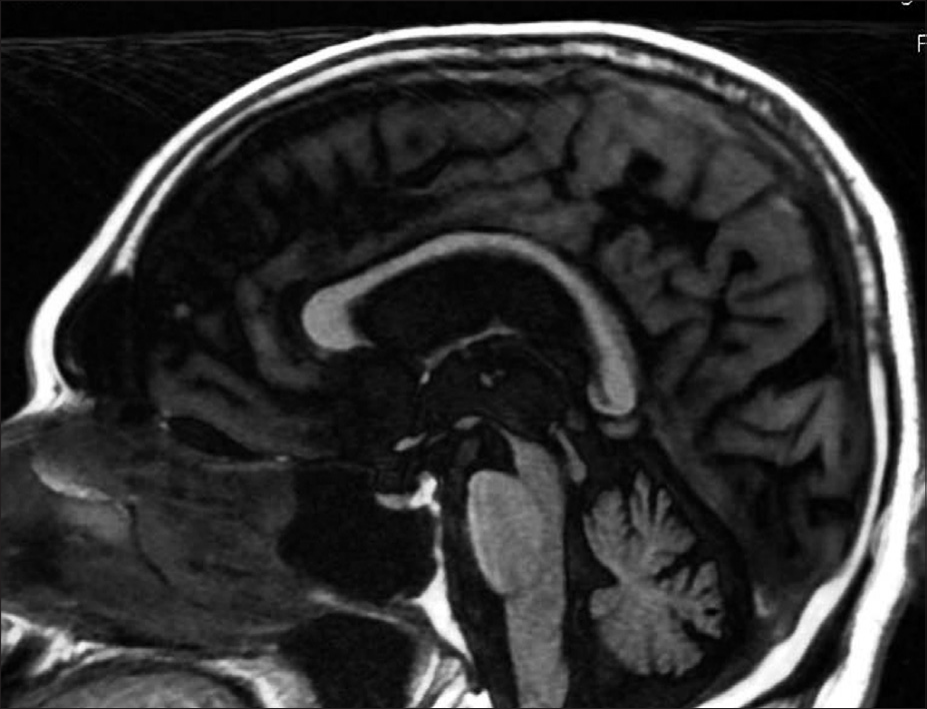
MRI brain T1W sagittal image showing atrophy of midbrain tegmentum – the humming bird sign in a 67-year-old man with progressive nonfluent aphasia and speech apraxia
